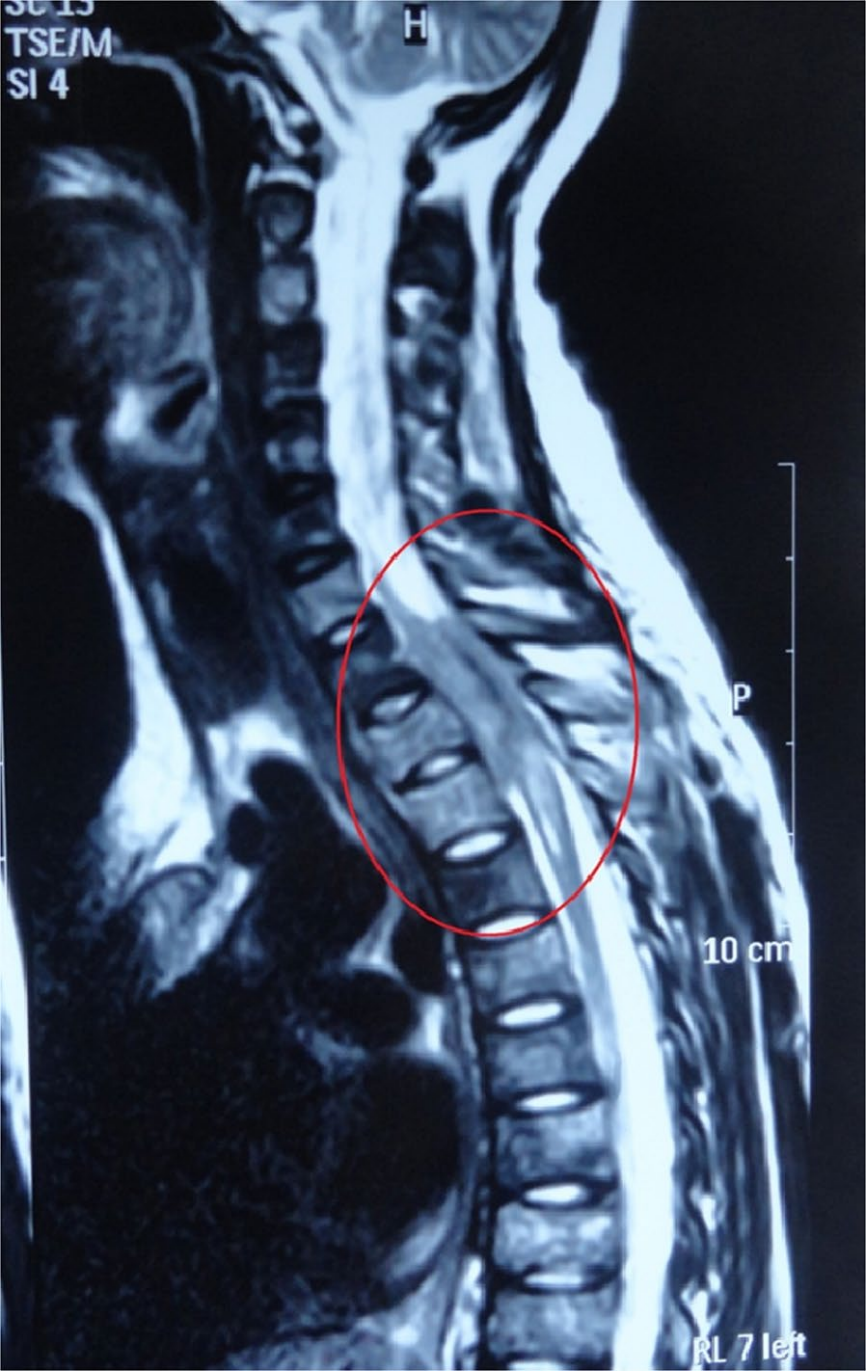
MRI image of cervical and thoracic spine. There is an intradural extra-medullary mass with an extraspinal component at C7-D2 level causing cord compression and there is a paraspinal component at this level which shows some degree of enhancement. Intervening intervertebral discs are normal.
